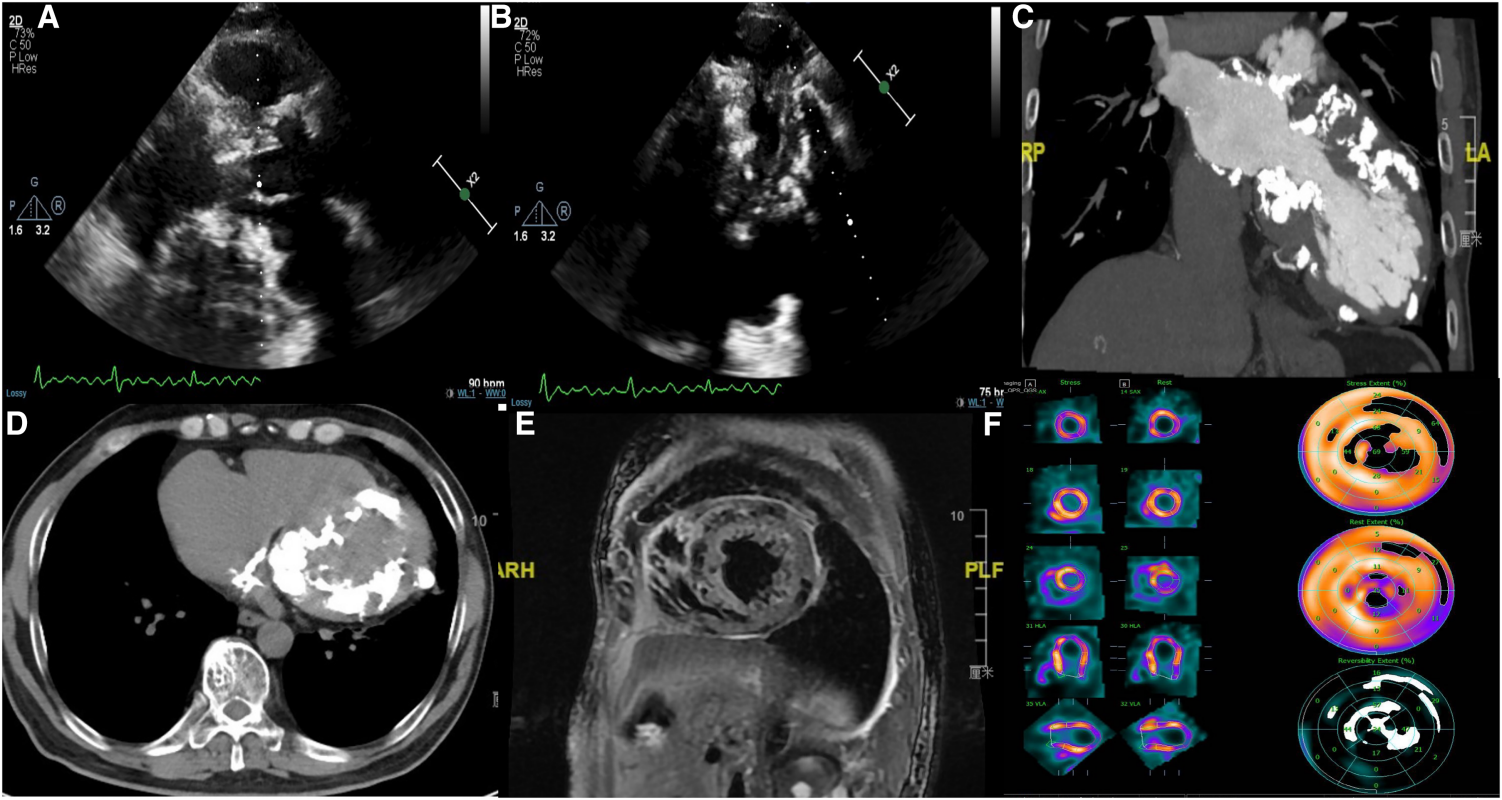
(A,B) Transthoracic echocardiography showing interventricular septal hypertrophy, extensive calcification in the left atrial wall, left ventricle, mitral annulus, and aortic wall; (C,D) cardiac computed tomography showing extensive calcification in the left ventricle, left atrium, atrial septa, and mitral annulus; (E) cardiac magnetic resonance imaging showing diffuse myocardial calcification; (F) radionuclide myocardial perfusion imaging (stress/rest) showing fixed perfusion defects.
